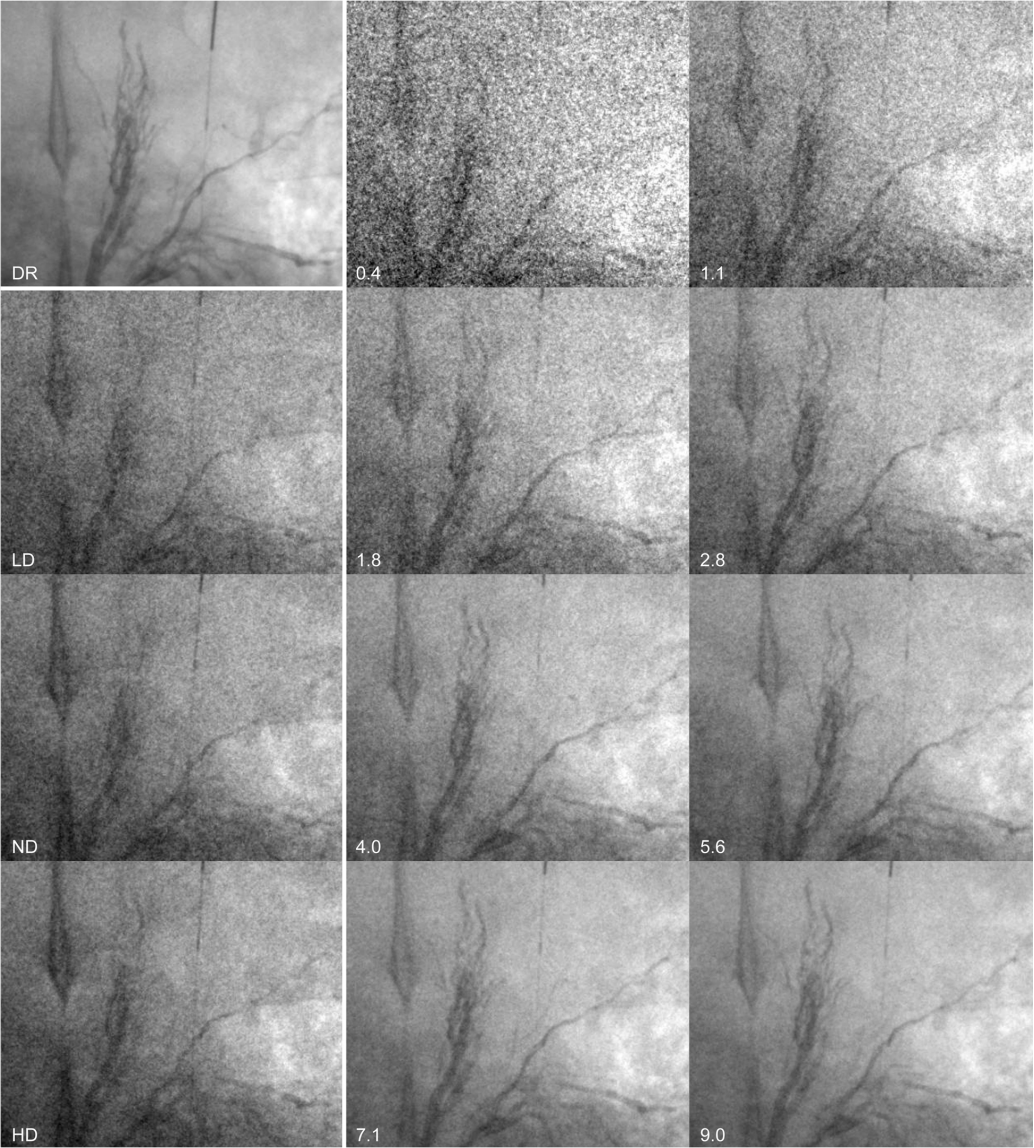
Images of Onyx® embolised liver and gastric arteries at 27 cm water equivalent thickness acquired with digital radiography (DR) as reference image, DEC using the three radiation dose levels (LD Low dose, ND Normal dose, HD High dose) and CEC using different image quality levels (IQ 0.4–9.0)
